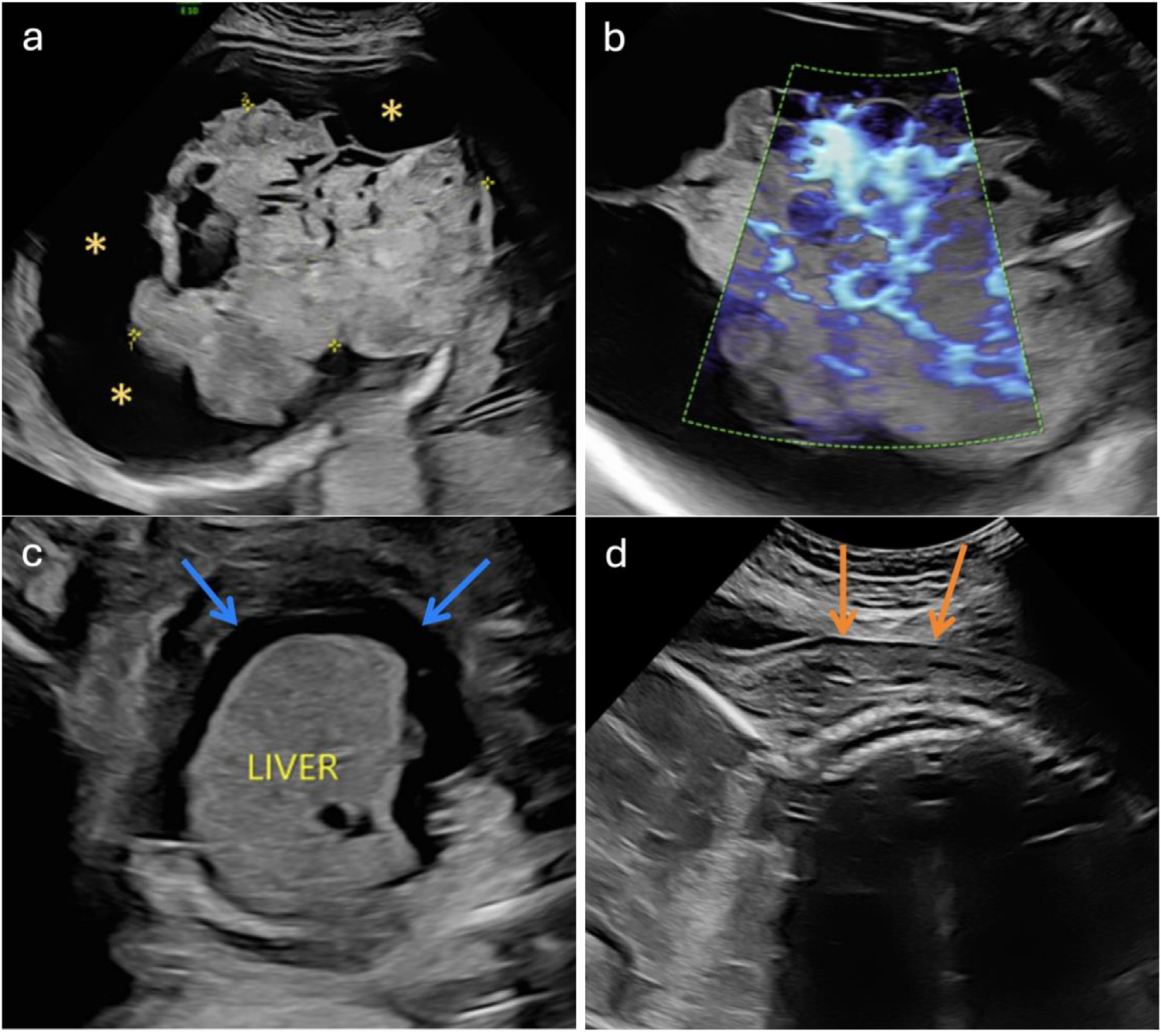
Follow-up sonogram at 29 weeks and 1 day. Transverse images demonstrate the size of the tumor, now measuring 12.59 × 8.79 cm (A), and the increase in vascularity (B), predisposing the fetus to high output cardiac failure. The cortex is severely thinned, making it difficult to visualize. There is significant fluid accumulation around the teratoma (A, asterisks). Hydrops of the fetus is observed with ascites (C, blue arrows) in the transverse plane, and skin edema (D, orange arrows) in the sagittal plane.
